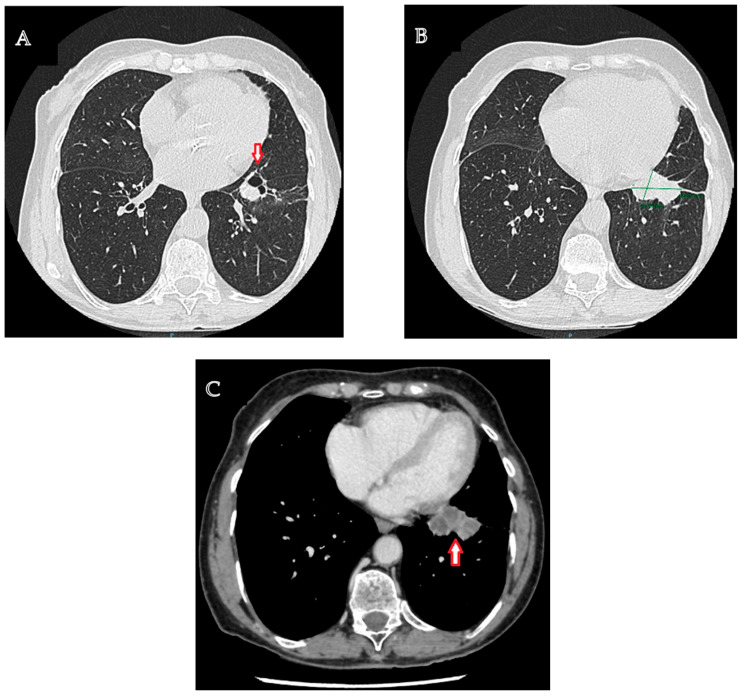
Typical carcinoid tumor located in the lower left lobe. (A). Contrast-enhanced chest computed tomography: a multiseptate lung tumor with multicystic appearance, located in the lower left lobe, associated with bronchial dilatations and discrete ground-glass areas. (B). Contrast-enhanced chest computed tomography: a lung tumor with maximum dimensions of 41/24/30 mm located in the lower left lobe. (C). Contrast-enhanced chest computed tomography, venous phase: a multiseptate lung tumor with multicystic appearance, located in the lower left lobe.
